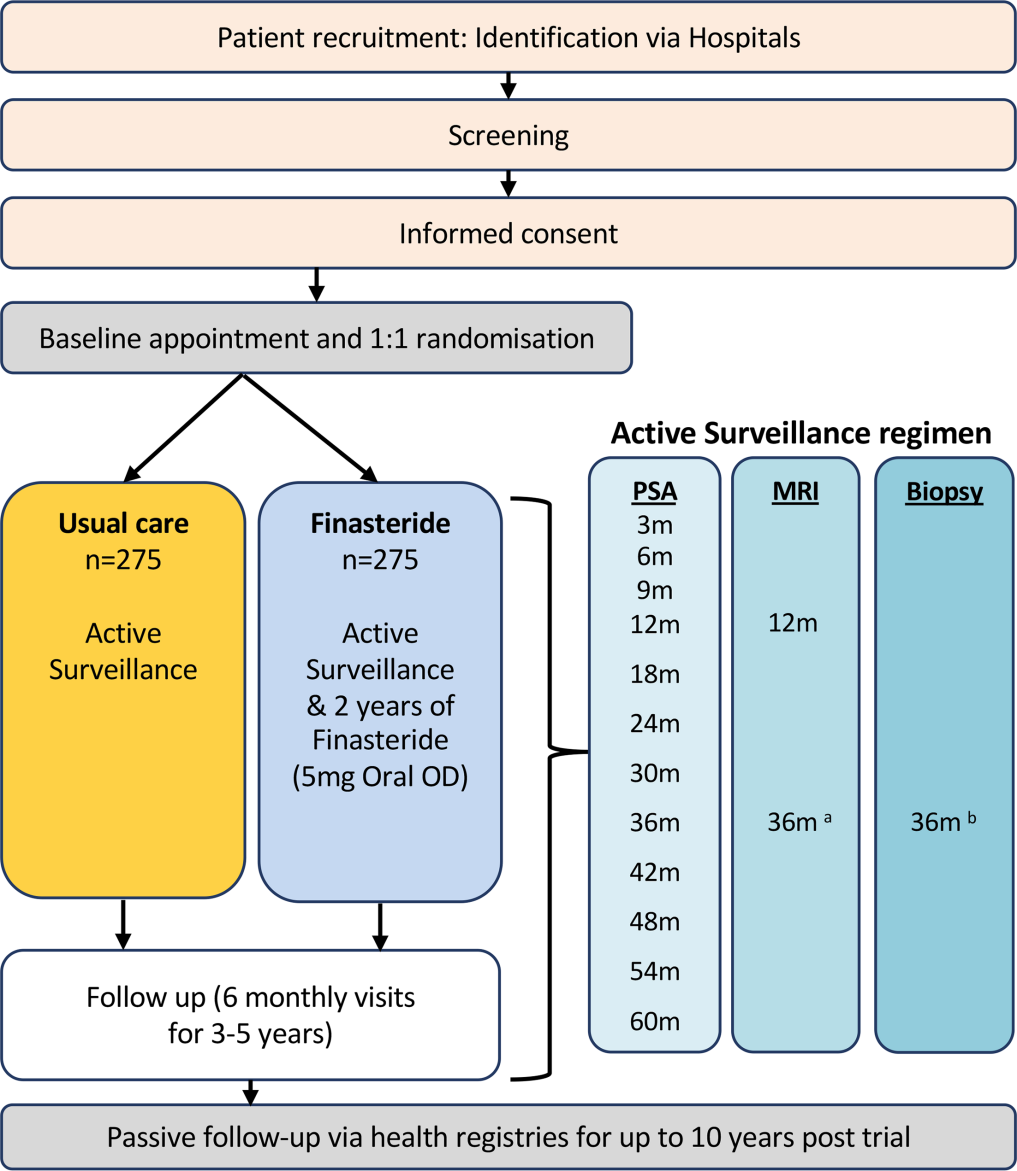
Recruitment and participant flow within the Finasteride in Active Surveillance for men with low and intermediate-risk prostate cancer (FINESSE) study. Follow-up within active surveillance includes PSA testing, MRI scans and the offer of a repeat biopsy (times in months (m) shown). ^a.^ Strongly recommended. ^b.^ Offered as a routine to all men. Also strongly recommended for changing MRI appearance and/or where indicated by the MRI scan. PSA, prostate-specific antigen.
